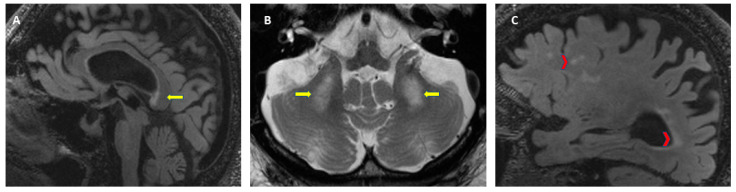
Neuroradiological criteria for the diagnosis of FXTAS. (A) T2-FLAIR: white matter lesions in the splenium of the corpus callosum, (B) T2-TSE: symmetrical white matter lesions in the middle cerebellar peduncles (MCP sign), (C) T2-FLAIR: cerebral white matter lesions and brain atrophy.
